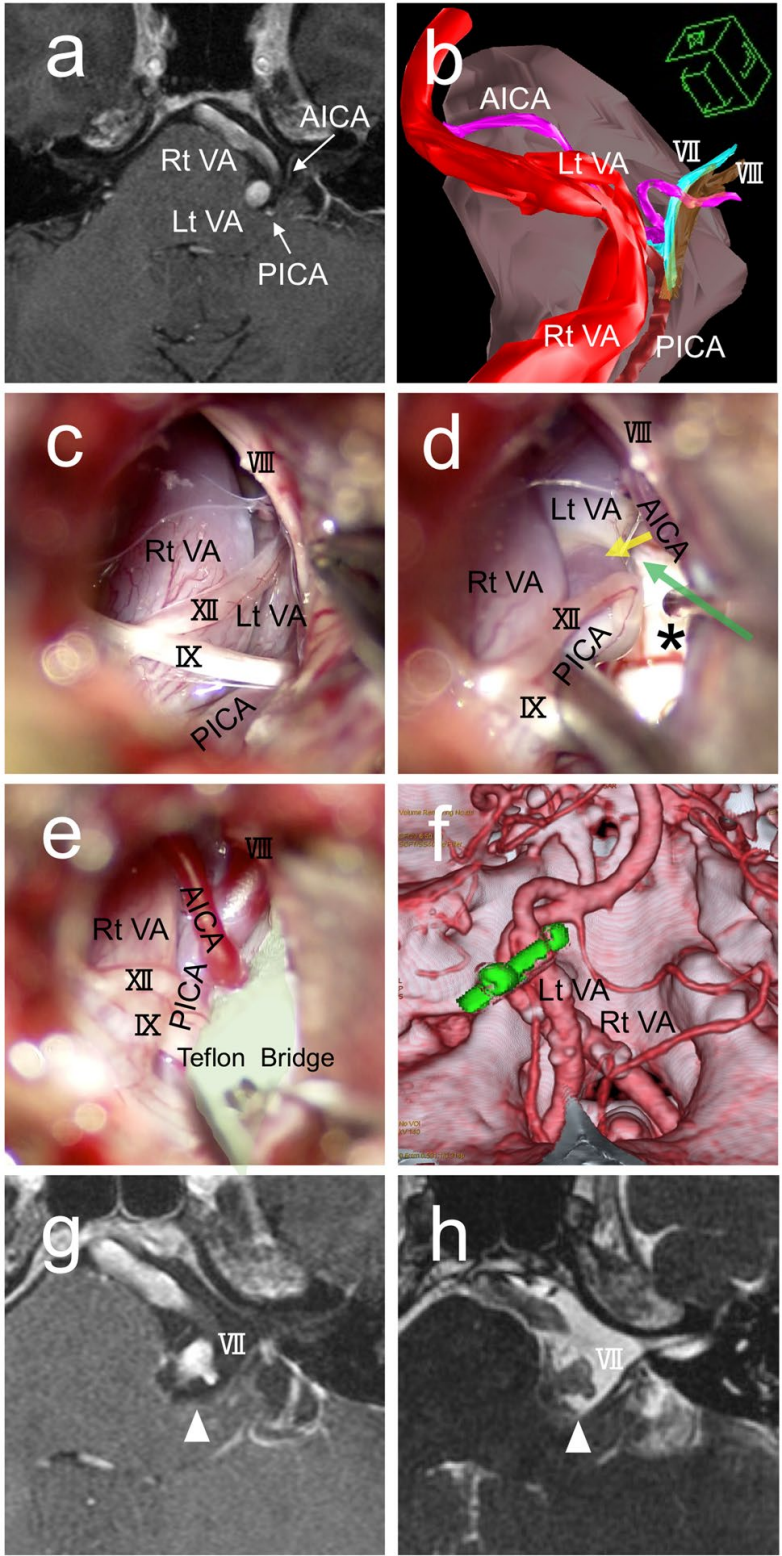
Case presentation (Case 3). A 44-year-old man with HFS on the left. The VAs on both sides, the PICA, and the AICA are involved. a A Gadolinium-enhanced MRI shows multiple offenders present near the REZ of the facial nerve on the left side. b A three-dimensional (3D) image looking from the lower-left clarifies the anatomical relationships of the offending arteries and the facial and vestibular nerves. c An intra-operative photograph shows the cistern is occupied with the offenders and the stretched cranial nerves. The hypoglossal nerve (XII) is at an unusual location stretched by the PICA orifice from the left VA. d A photo showing identification of the REZ of the facial nerve (black asterisk) after elevating both vertebral arteries and the PICA away from the brainstem. The AICA is still deeply impinging onto the REZ of the facial nerve. The AICA is relocated laterally (yellow arrow) together with other offenders, and then, a Teflon bridge is inserted into the gap (green arrow). e All offenders are held with a Teflon bridge (colored in light green). Two additional small Teflon pieces are placed on both edges to secure the position of the bridge. f A post-operative 3D-CT demonstrates the bridge (green) is holding all offenders. g An enhanced MRI shows the offenders elevated away from the facial nerve with a free space over the REZ (white arrowhead). h An MRI with fast imaging employing steady-state acquisition shows cerebrospinal intensity over the REZ of the facial nerve (white arrowhead)
